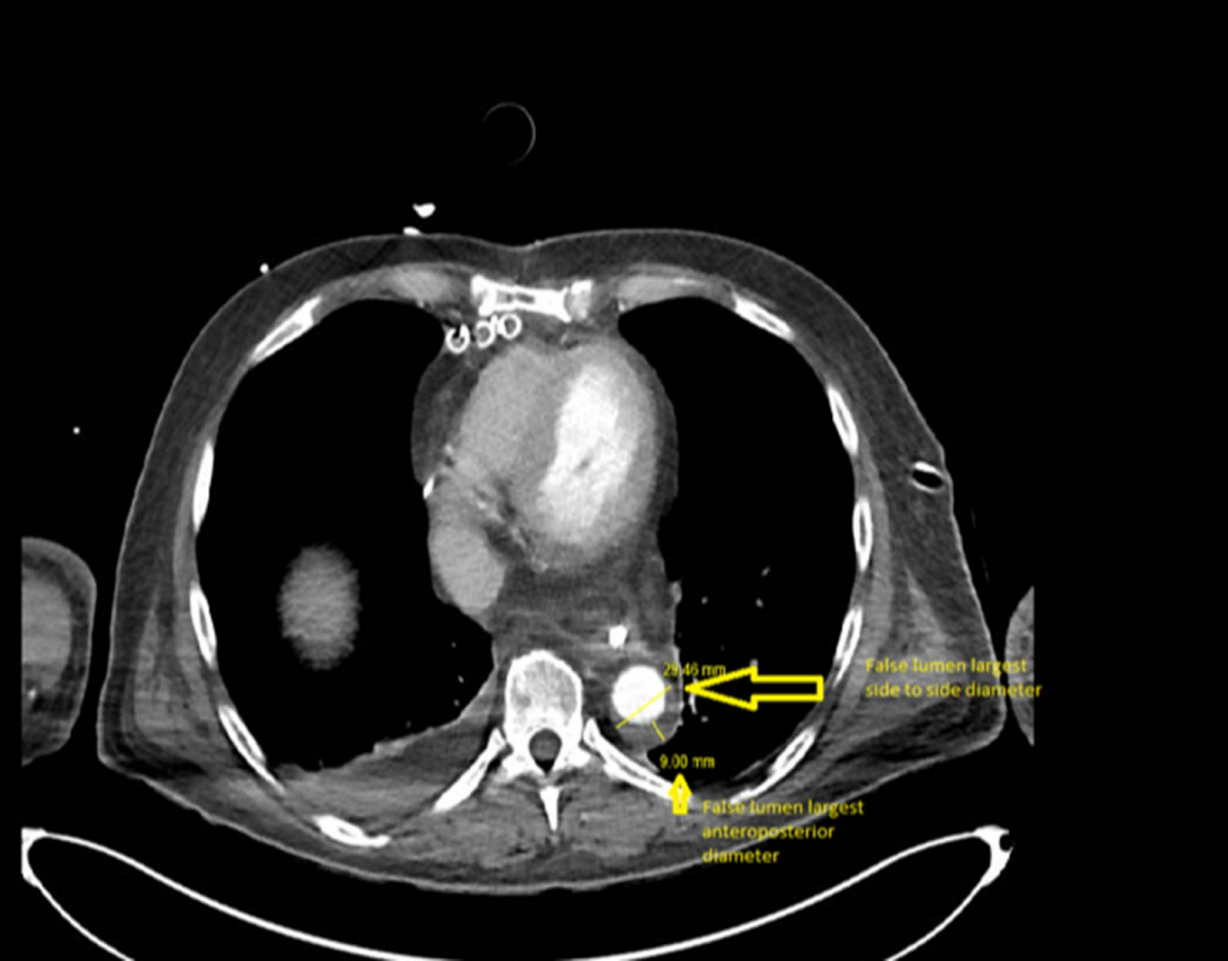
Multislice computed tomography showing false lumen largest anteroposterior and side to side diameters at the level of the right diaphragmatic dome
